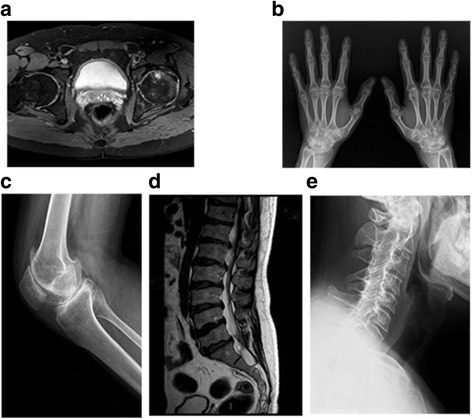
Radiographs of the proband at the age of 35 years. a Reduced interarticular spaces in the hip joints with bilateral femoral neck aseptic necrosis, b enlargement of interphalangeal joints, c narrowing of elbow joint space, d osteosclerosis and an irregular contour of the joint surface and narrow spaces in the knee joints, e herniated disks in the thoracolumbar spine (T12/L1-L5/S1) with lumbar spine hyperplasia, (f) and flattened vertebral bodies in the cervical spine can be noted
